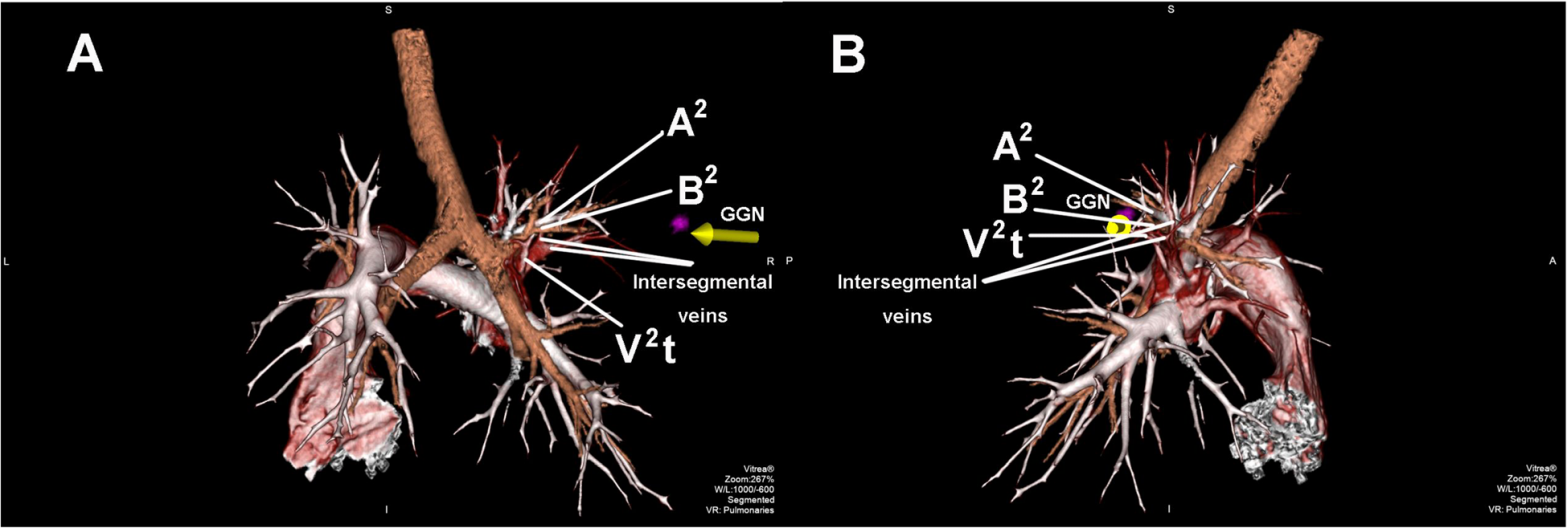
The three-dimensional computed tomography bronchography and angiography. The apical subsegmental bronchi(B^1^a and B^1^b) originated from the posterior segmental bronchus (B^2^) and the anterior segmental bronchus (B^3)^ respectively; The right upper pulmonary artery shared trunk without posterior ascending artery (Asc.A^2^); The right upper pulmonary vein had no central vein, with only one posterior intrasegmental vein(V^2^t), the other two veins pointed to the intersegmental plane respectively. a Posterior view; b Lateral view
